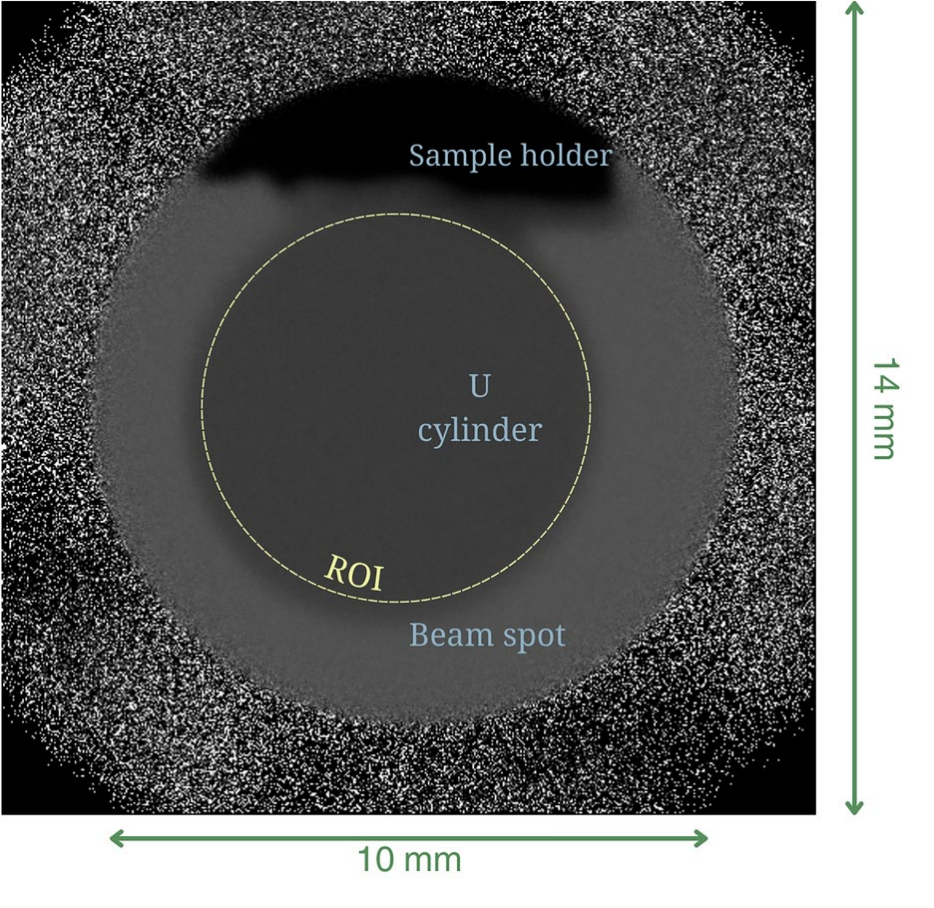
Thermal neutron radiograph of a 5 mm thick depleted uranium cylinder resulting from ten minutes count times. The dashed circle indicates the ROI used for analysis. The beam spot is 10 mm in diameter, and the FOV is 14 × 14 mm^2^.
